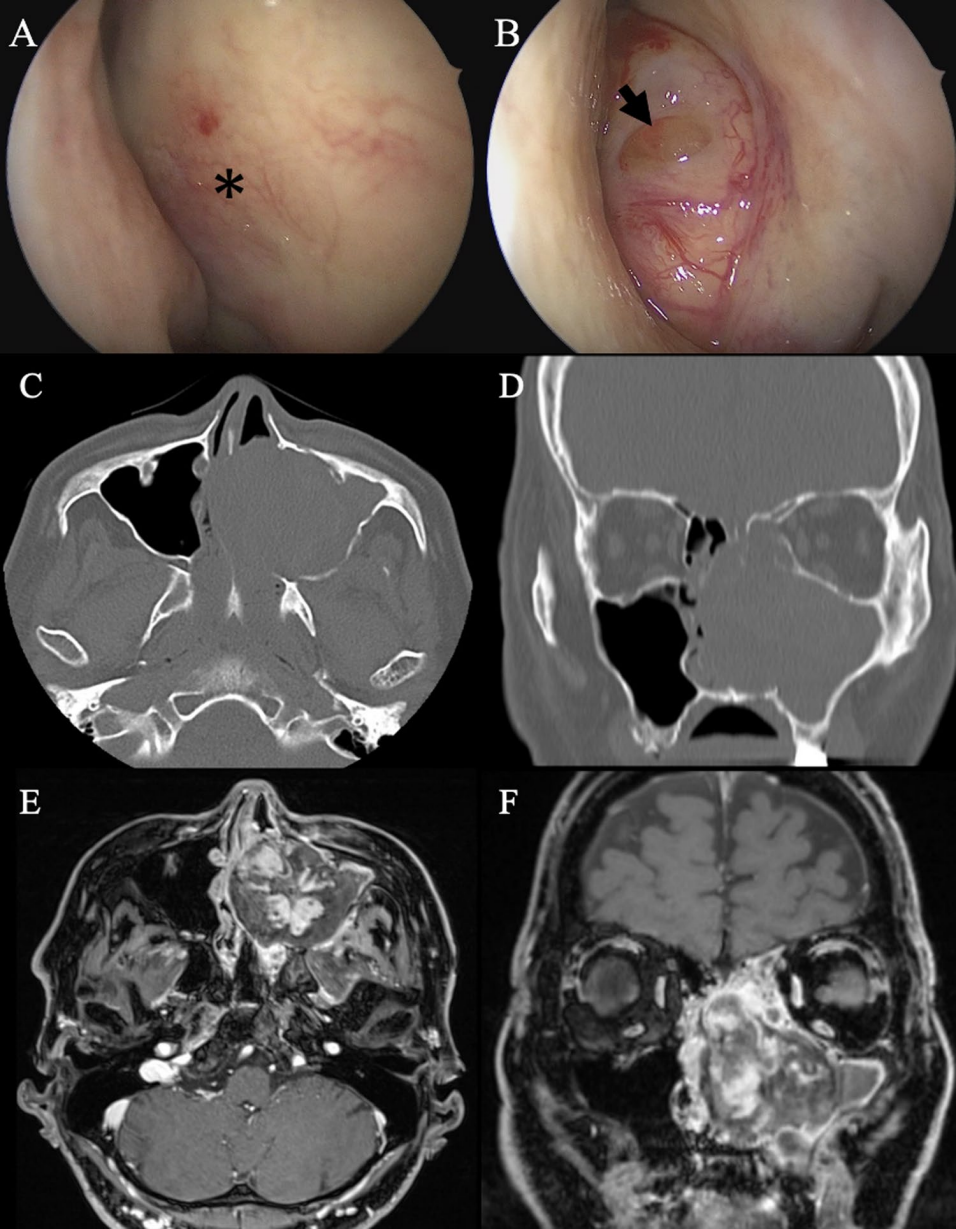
A Endoscopic view of right nasal cavity. The right nasal cavity was obstructed due to compression of the nasal septum: asterisk. B Endoscopic view of the lesion partially revealed a smooth, polypoid mass arising from the natural ostium of the left maxillary sinus: black arrow. Axial (C) and coronal (D) CT showing a soft-tissue, isodense mass in the peripheral area occupying the left nasal cavity. Multiple bony structures eroded by growth including the medial wall of the right maxillary sinus and a large portion of the nasal septum are visible. Axial (E) and coronal (F) MRI showing oedematous mucosa surrounding a heterogeneous tumor in the left nasal cavity
